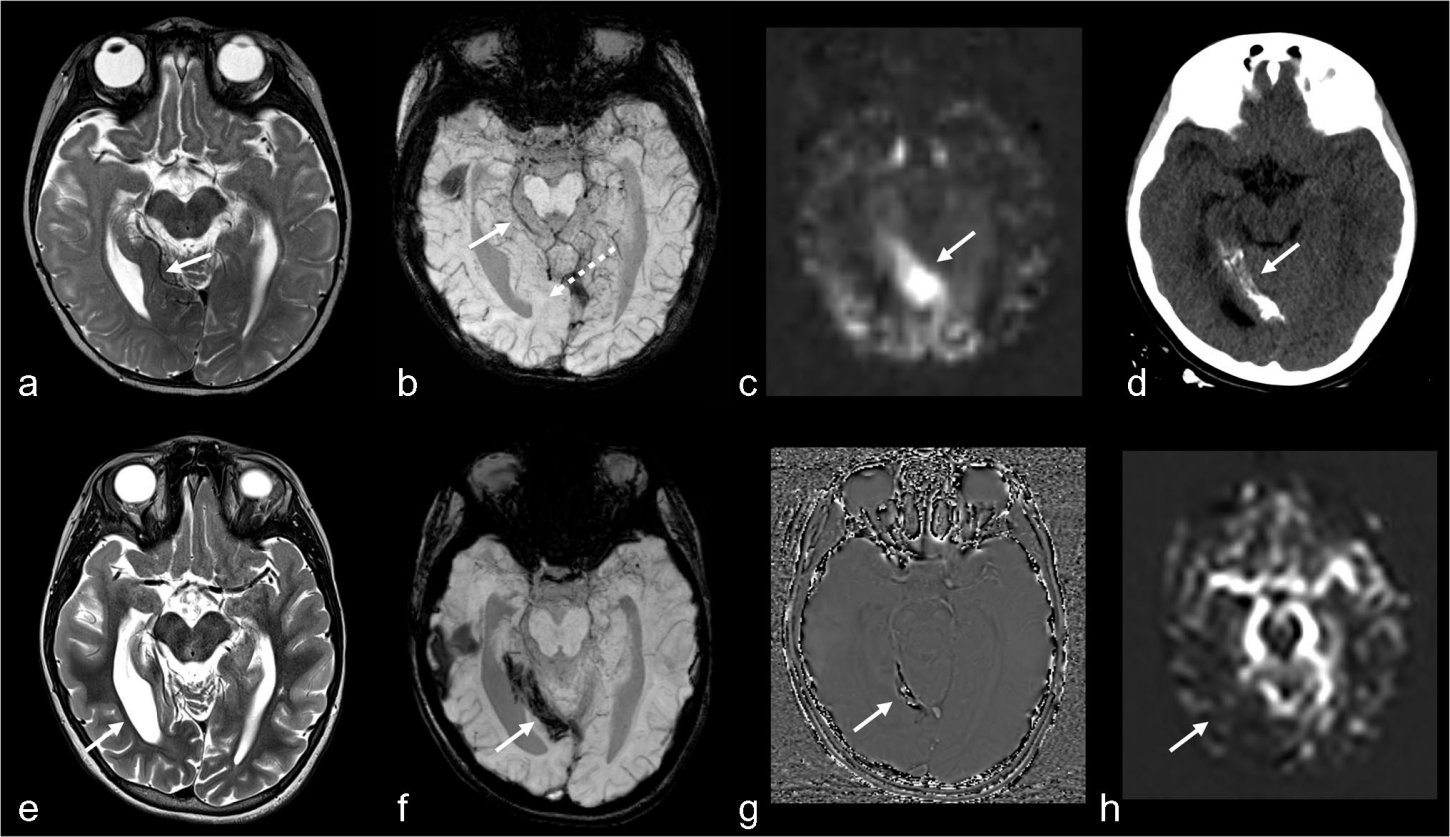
Perfusion changes and evolution to tissue necrosis in a girl presenting with status epilepticus. Magnetic resonance imaging (MRI) at 6 months old (a-c). Axial T2WI (a) shows enlarged deep occipital veins tributaries to the internal cerebral vein and vein of Galen. (arrow) without significant parenchymal changes. Axial susceptibility-weighted imaging in minimum intensity projection (SWI MinIP) (b) confirms the presence prominent deep veins (solid arrow) and absent cortical veins in the calcarine region (dashed arrow). ASL perfusion (c) shows increased perfusion in the medial calcarine aspect of the right occipital lobe (arrow) where there was a lack of cortical veins, indicating venous congestion. Head CT without contrast (d) performed at 7 years of age, shows interval development of calcifications, involving in the middle calcarine aspect of the right occipital lobe that show the characteristic tram-track appearance (arrow). Follow-up at MRI at 8-years-of- age (e–h) Axial T2 (e) shows cortical atrophy with secondary enlargement of the right occipital horn (*arrow*). Axial SWI MinIP (f) demonstrates coarse susceptibility artifacts (*arrow*), in keeping with known subcortical calcifications, (g) Phase imaging demonstrates signal changes corresponding to calcifications. ASL perfusion (h) shows areas of decreased perfusion (arrows), indicating tissue necrosis. Note the hyperperfused (and later hypo perfused areas) matched the location of SWS findings on conventional imaging
